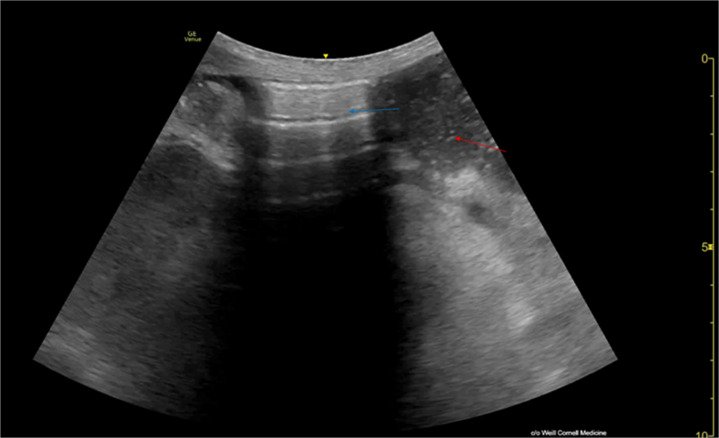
Abdominal point of care ultrasound (POCUS) with patient in the supine position. A convex transducer in transverse orientation in the abdominal preset (2.0 MHz) is used. The image suggests dilated bowel loops compatible with intestinal obstruction; the red arrow indicates a fluid and gas-filled dilated loop. The blue arrow indicates air in the bowl. The presence of A-lines mimics pneumoperitoneum. The image is courtesy of Tanping Wong, MD of Weill Cornell Medicine.
